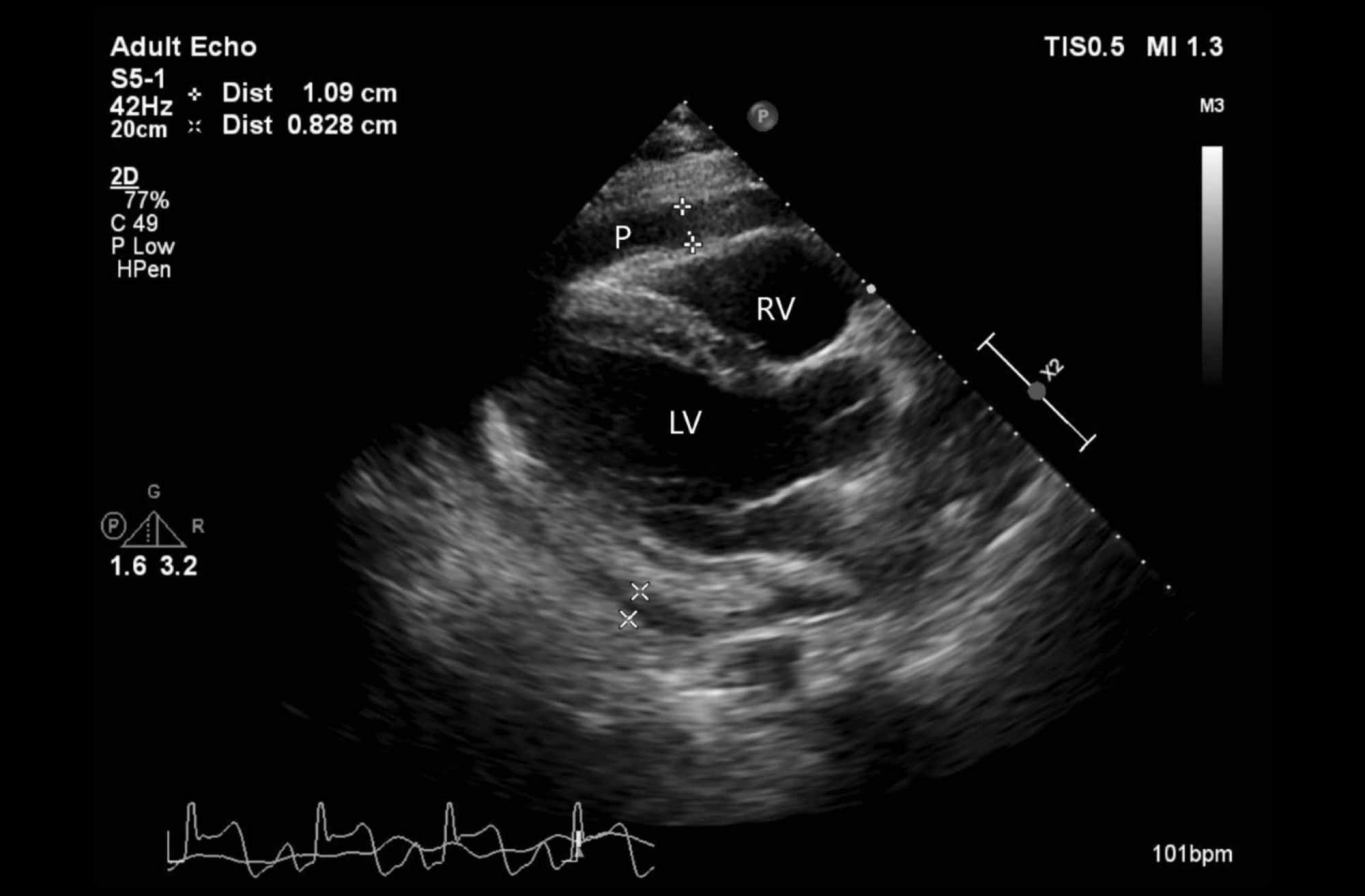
Parasternal long axis of transthoracic echocardiogram (TTE) Parasternal long axis view of TTE demonstrates circumferential effusion in pericardial space (P). LV, left ventricle. RV, right ventricle.
